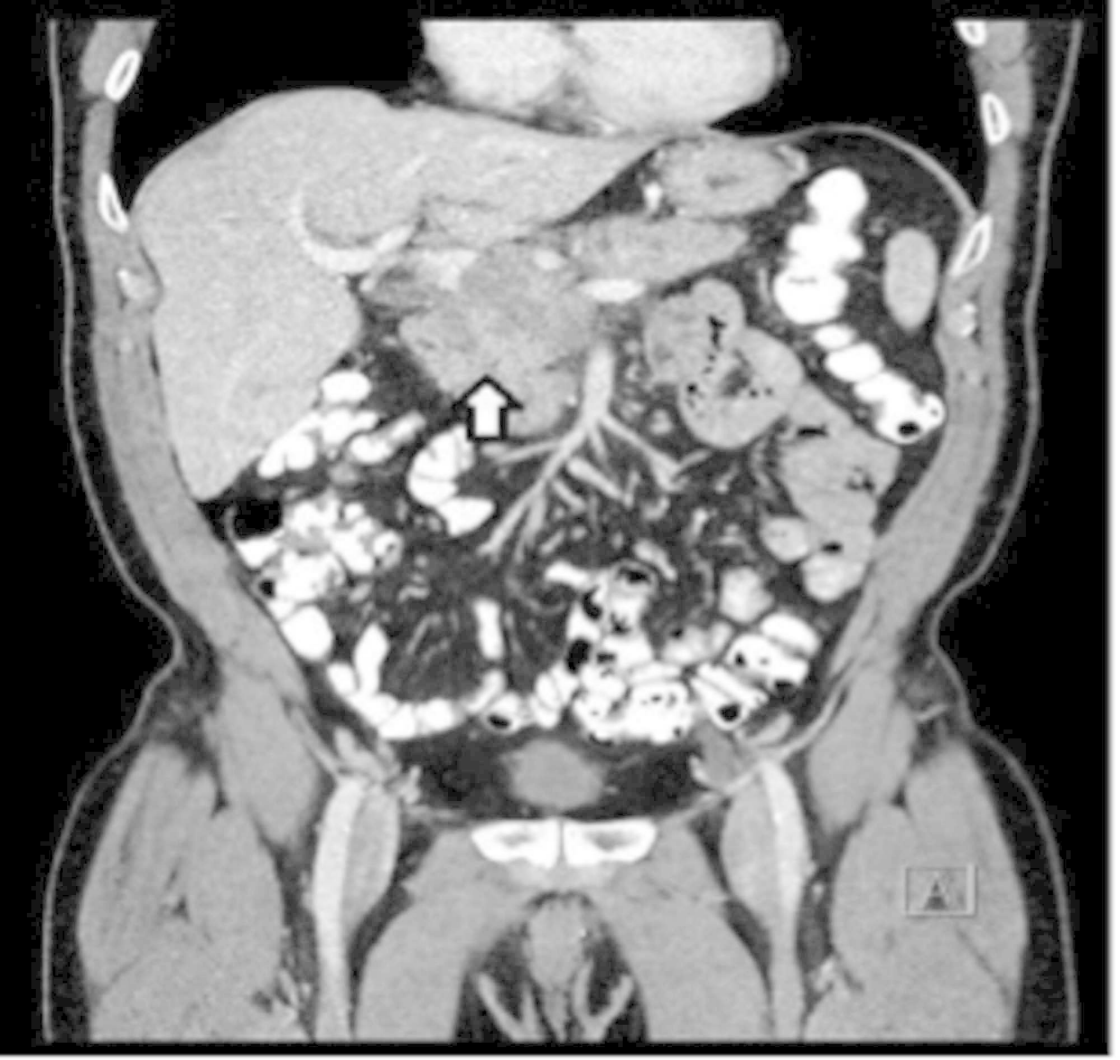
CT abdomen/pelvis showing interval development of an infiltrative pancreatic head mass (white arrow) s/p six cycles of chemotherapy. CT: Computed Tomography, s/p: Status Post
